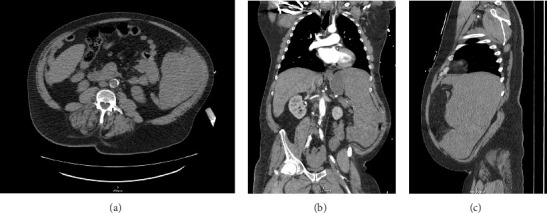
Computed tomography angiogram of the chest, abdomen, and pelvis in the (A) transverse, (B) coronal, and (C) sagittal planes, demonstrating enlarged spleen with large adjacent hematoma extending into the patient's pelvis.
